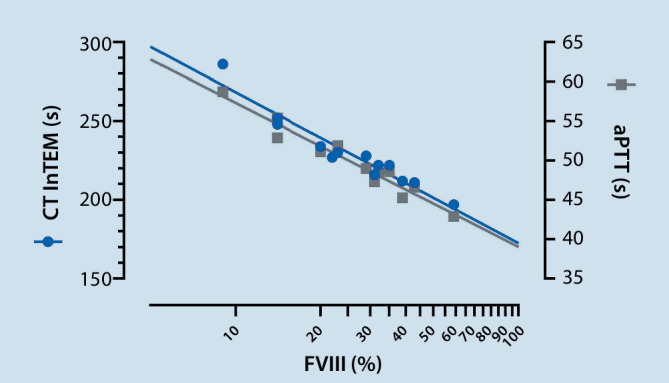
The pharmacokinetic profile of the patient was established prior to surgery. The InTEM clotting time (CT InTEM) and aPTT were measured to predict FVIII activity (FVIII). Depending on the intraoperative results of the CT InTEM, efmoroctocog alfa was substituted accordingly
